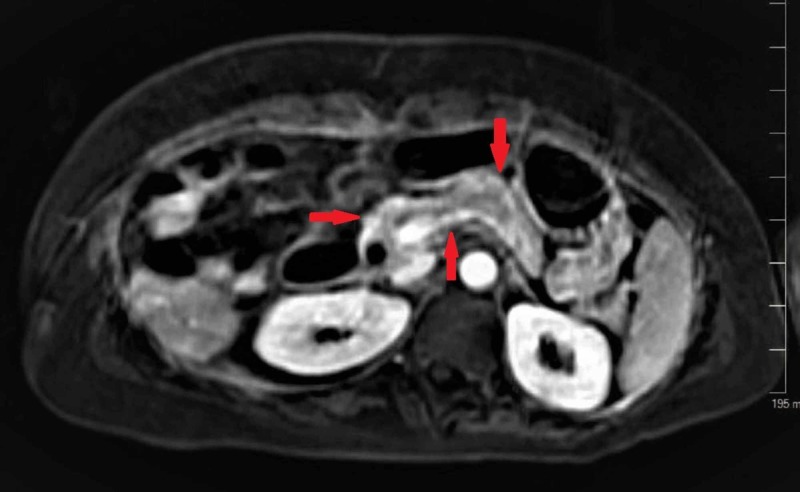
MRI of the abdomen with contrast showing the normal pancreas without any pancreatitis or pancreatic lesions.
